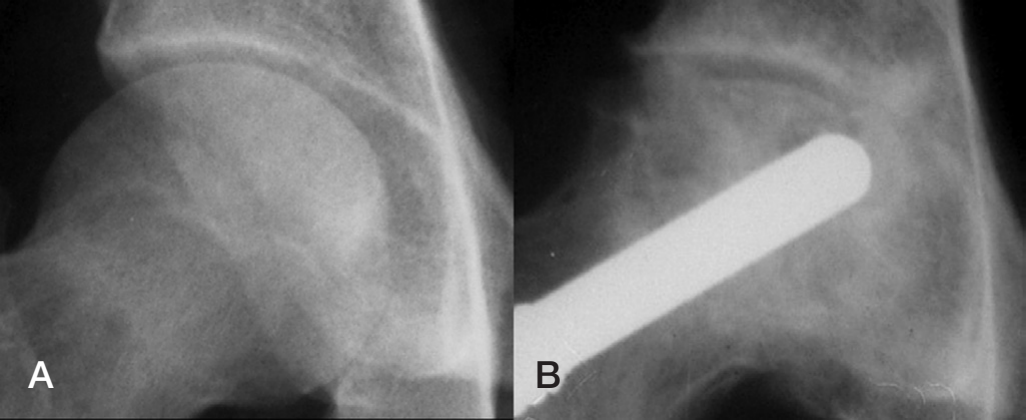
27-year-old man with osteonecrosis secondary to cortisone intake for Hodgkin lymphoma. A. Preoperatively, the disease was clas-sified as Steinberg stage II. B. 3 years later, the hip had deteriorated radiographically to Steinberg stage VI and required revision to THA.
